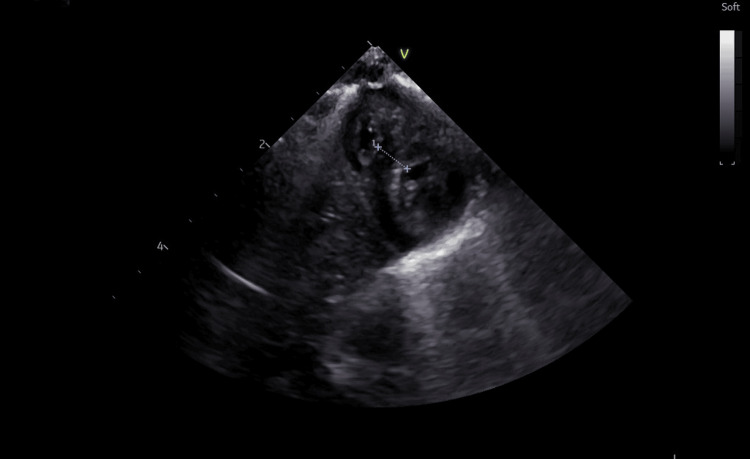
Echocardiography: short-axis view of the left ventricle showing left ventricle hypertrophy
